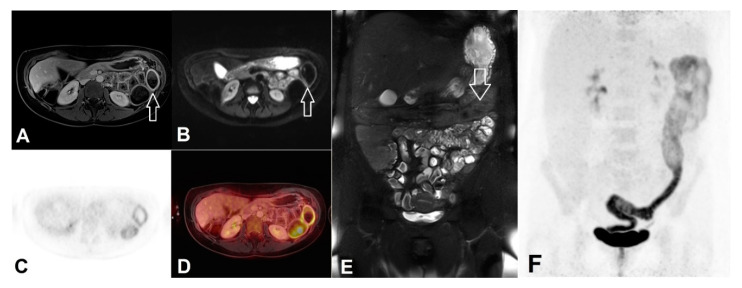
Left-sided colitis in a female patient without previous bowel purgation. Despite feces in colon, left-sided colitis could be found in all PET/MR modalities as well in the ileocolonoscopy. (A) Intensive mucosal enhancement of contrast agent in left colon (white arrow) in T1w-3D-VIBE with fat saturation, (B) Hyperintensity of bowel wall shown in the *b*1000 s/mm^2^ of DWI, (C) PET, (D) Fused imaging of PET and MR, (E) Feces in the left-sided colon (white arrow) shown in the fat-saturated T2w HAST (F) Maximum intensity projection of PET demonstrating increased tracer uptake throughout the left-sided colon.
